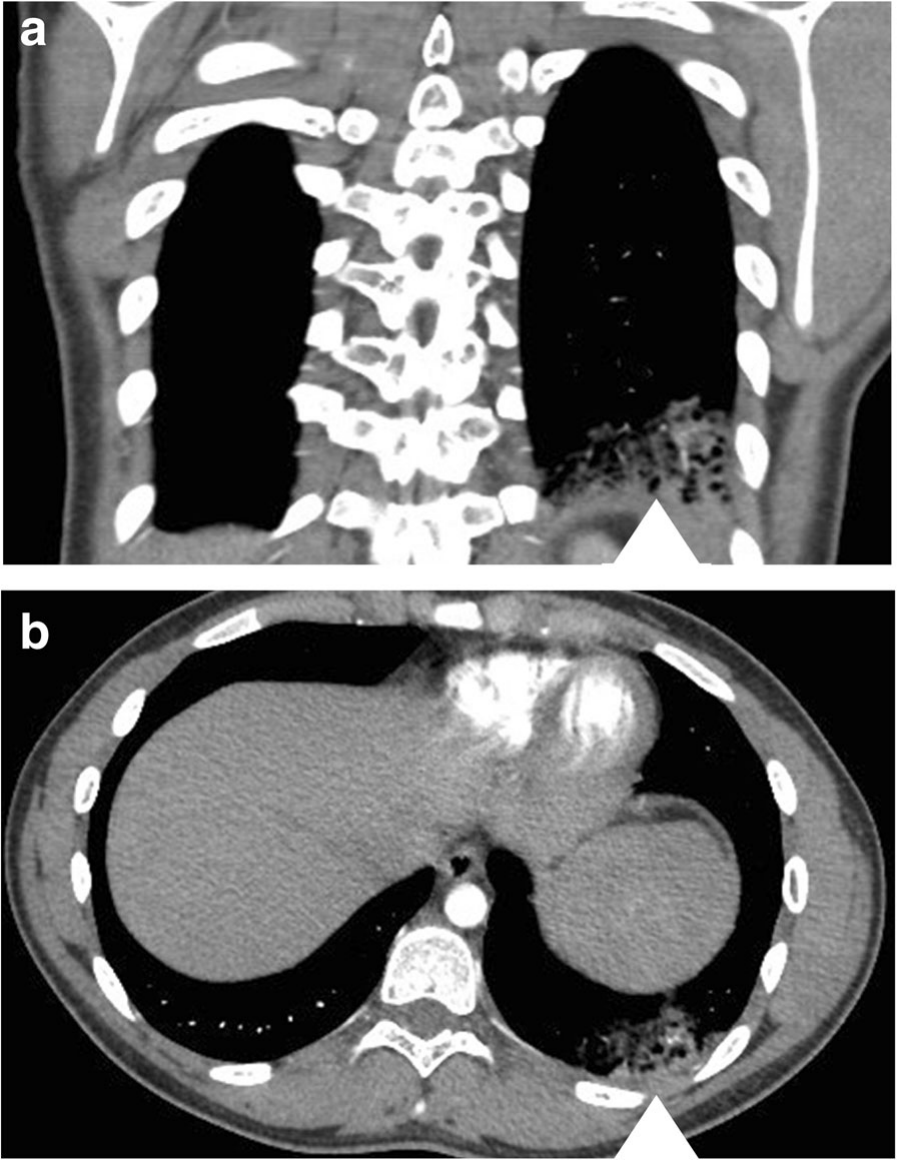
Bilateral lower lobe pulmonary embolism more numerous on the left. There is an associated opacity in the left lung base probably representing pulmonary hemorrhage/infarction. a Coronal and b axial views showing multiple filling defects in the left lower lobe pulmonary arteries as well as a groundless opacity (*white arrowhead*) compatible with pulmonary hemorrhage related to the pulmonary embolism. Hematology decided not to rescan with computed tomography to show resolution of the pulmonary embolism following 3 months of anticoagulation therapy
